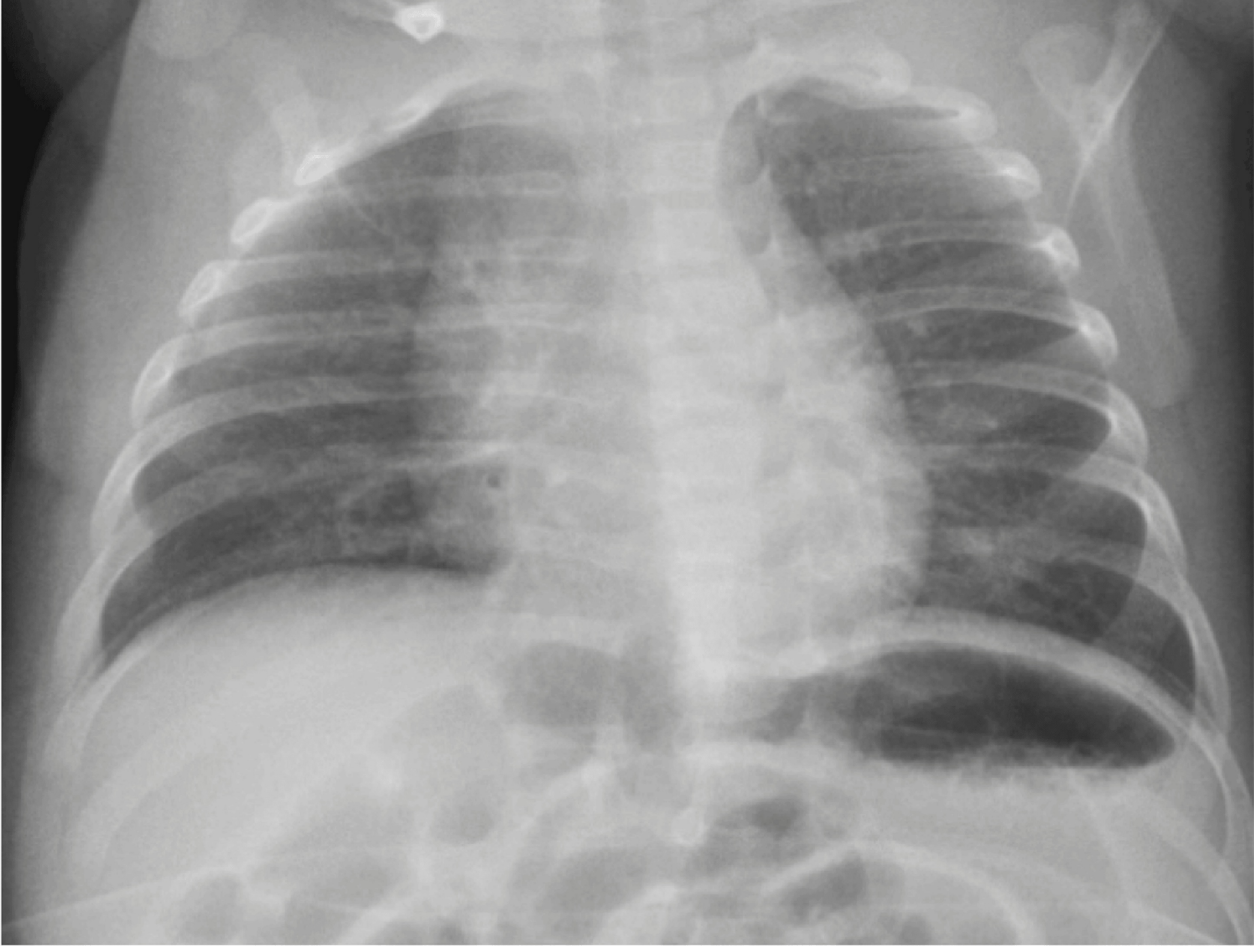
Chest x-ray showing a visible thymus with no mediastinal widening.
